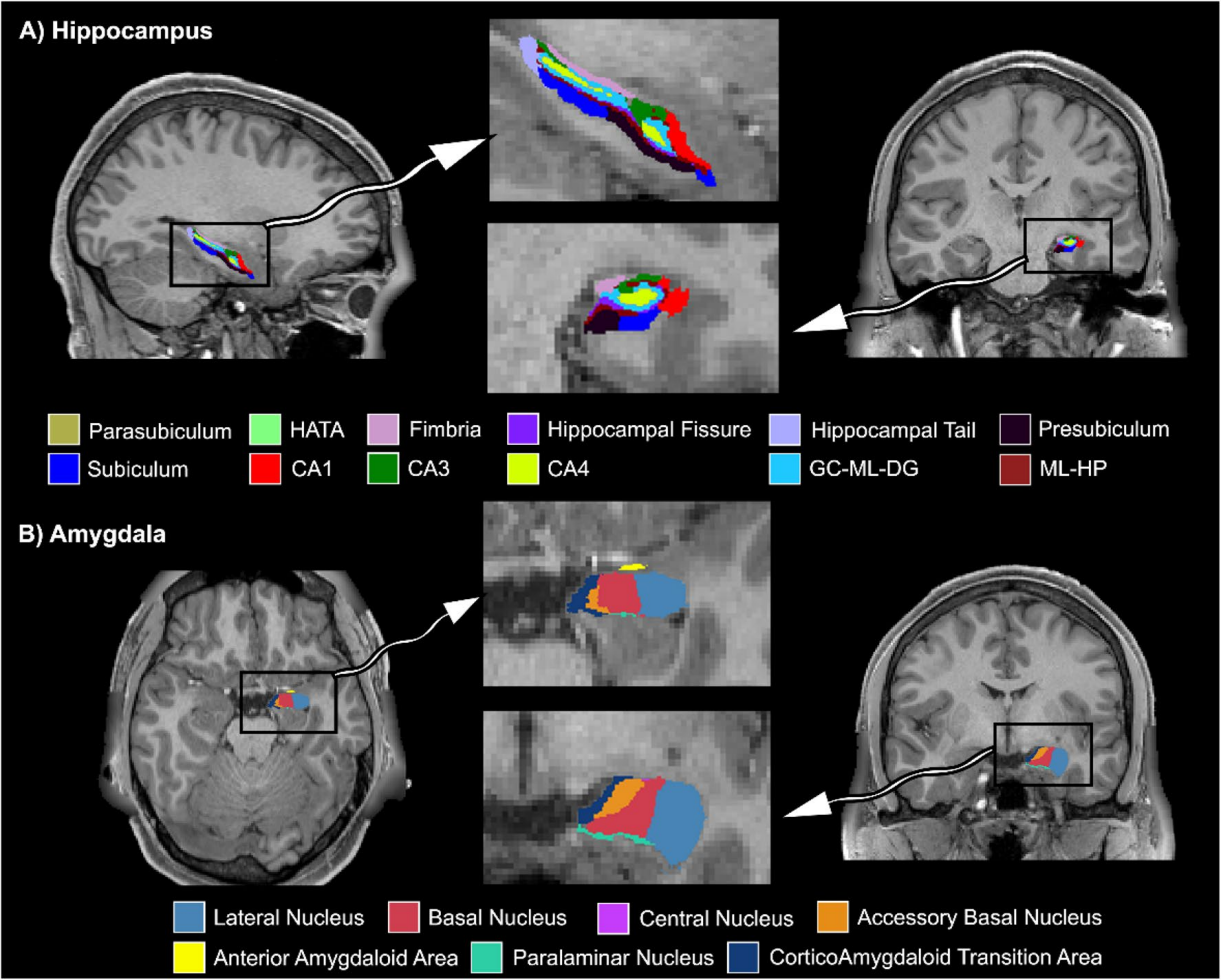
Left hippocampal subfields (A) and subnuclei of amygdala (B) in one of the subjects labeled on the T1 MRI scan. HATA: Hippocampo-amygdalar transition area, CA: Cornu Ammonis, GC-ML-DG: Granule cell and molecular layer of dentate gyrus, ML-HP: Molecular layer of hippocampus.
